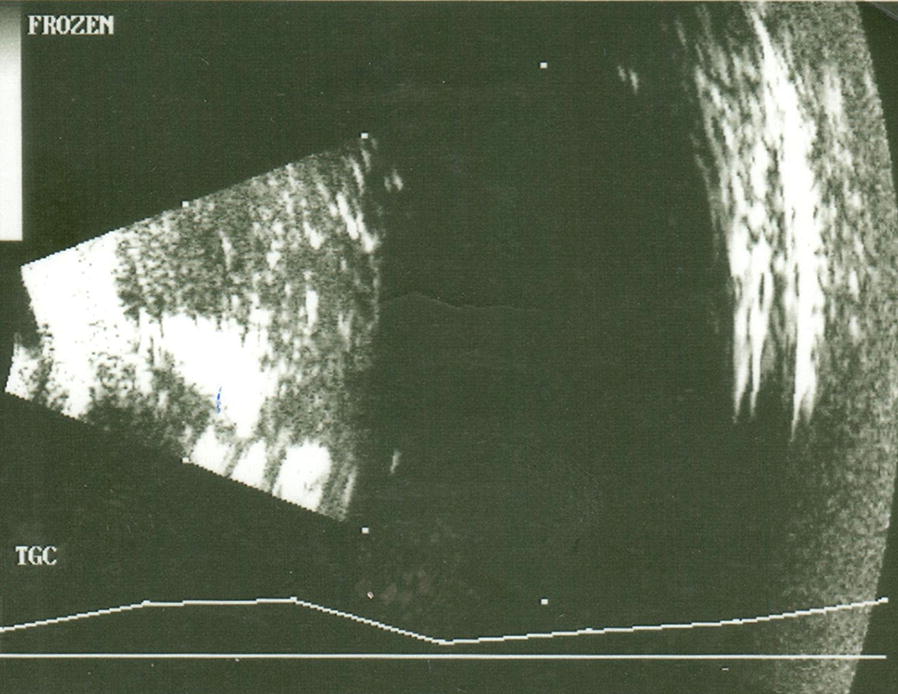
Case 1 B scan ultrasonography of the right eye done on the 11th of August before silicone oil removal, showing high echogenicity in the anterior vitreous cavity
